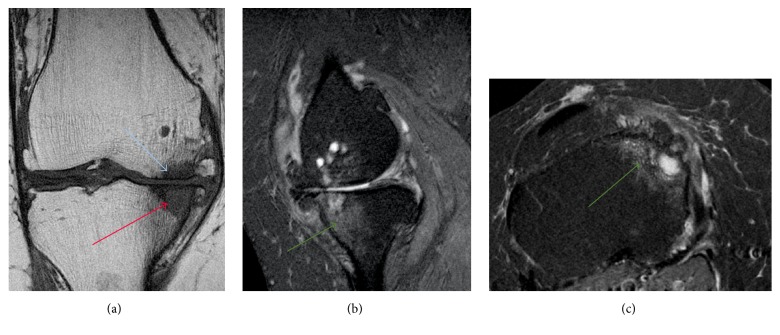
(a) Coronal T1 MRI with contrast image in painful knee OA patient showing large medial proximal tibial BML (red arrow) and smaller medial distal femoral BML (blue arrow). (b) Corresponding proton density weighted fat suppressed sagittal MRI sequence showing the large anteromedial BML (green arrow). (c) Axial proton density weighted fat suppressed MRI showing the tibia BML.
